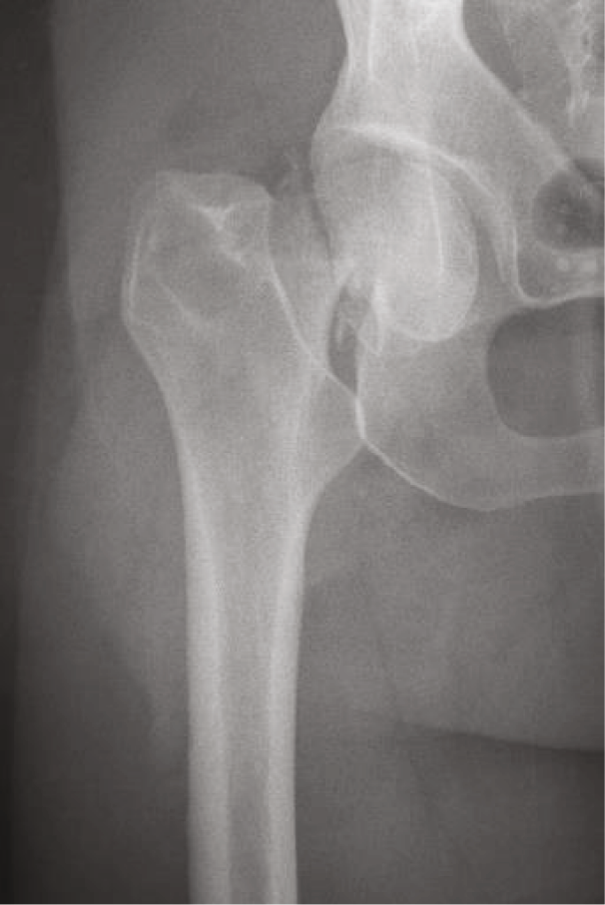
AP radiograph of the right hip demonstrating a subcapital femoral neck fracture.
